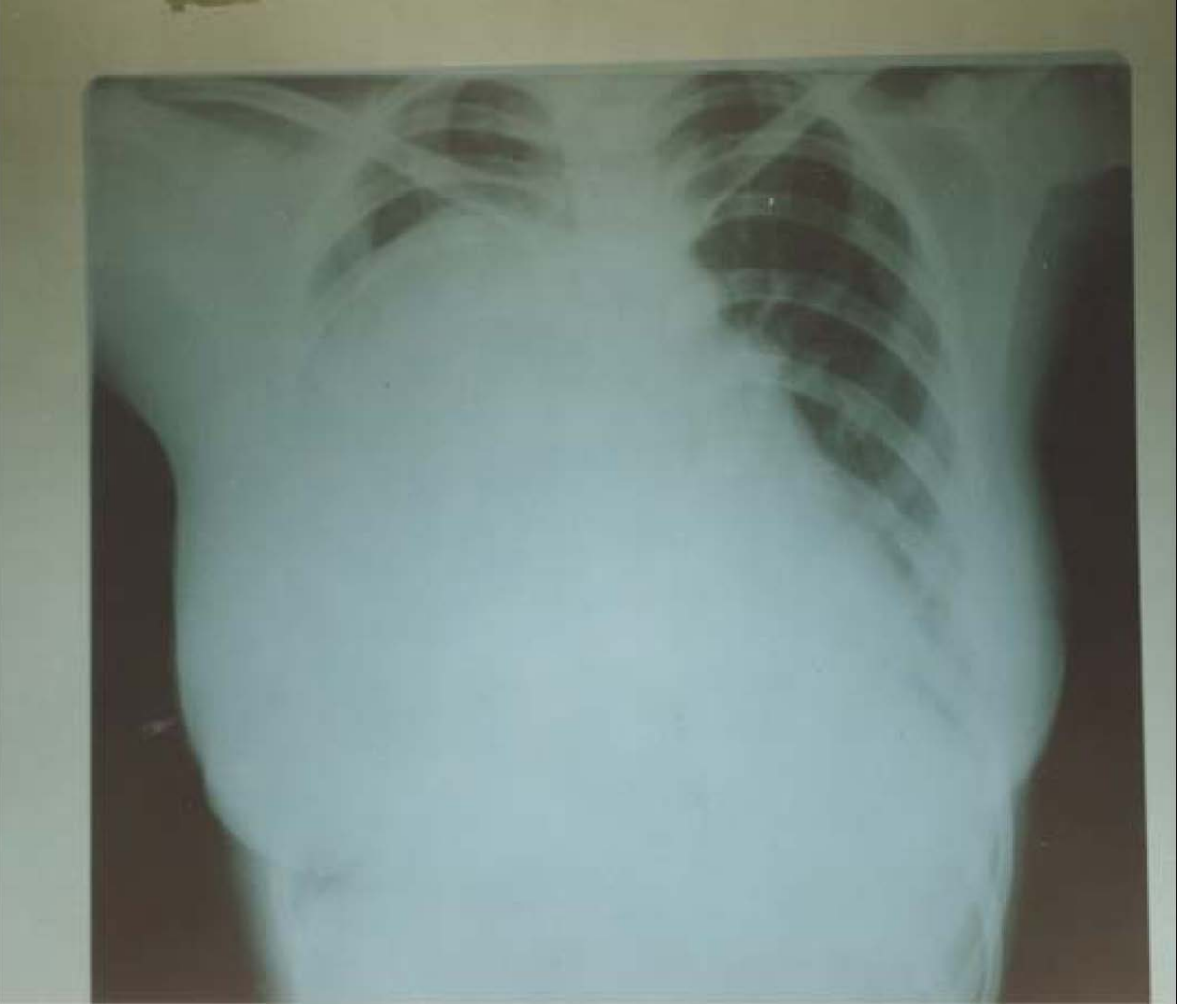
Chest X-ray showing a dense homogenous radiopaque opacity involving most of the right hemithorax.
